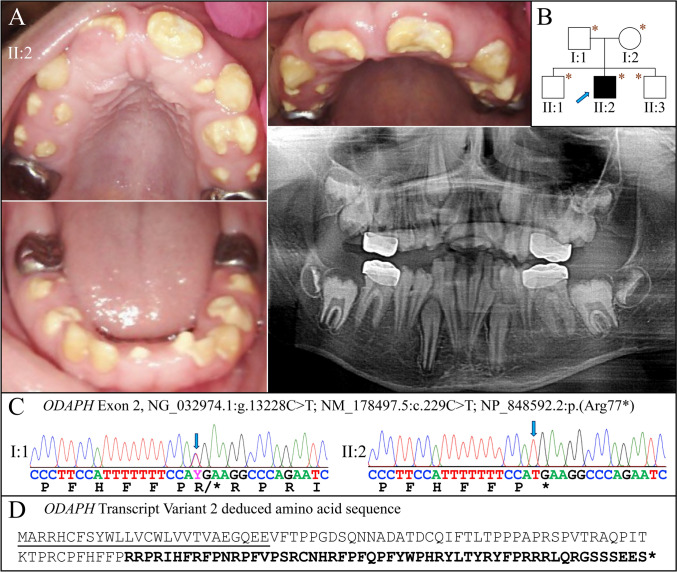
Hypomaturation Amelogenesis Imperfecta Caused by an *ODAPH* Homozygous Truncation Mutation (p.Arg77*). A Oral phenotype and panoramic radiograph of the male proband (II:2) at age 12.5 years. The permanent dentition is not fully erupted (delayed). The gingiva still partially covered the maxillary bicuspids. All four second molars failed to erupt, with the mandibular molars showing a small coronal radiolucency with a thin sclerotic border, indicating they had not yet emerged from the overlying alveolar bone. The enamel of unerupted teeth was slightly more radiopaque than dentin and underwent rapid attrition following eruption. B Both parents (I:1 and I:2) and the younger brother (II:3) were heterozygous for the same *ODAPH* variant (c.229C > T) that converted arginine codon 77 (CGA) into a STOP (TGA) codon. *Denotes study participants. Only the proband showed a detectable enamel phenotype. C DNA sequence chromatograms showing the father’s heterozygous *ODAPH* sequence and the proband’s (II:2) homozygous sequence for the variant that caused the AI phenotype (Table 1). D Amino acid sequence of *ODAPH* transcript variant 2 (NM_178497.5). The signal peptide that is cleaved after directing the protein into the secretory pathway is underlined and the amino acids lost due premature termination of translation are in bold
